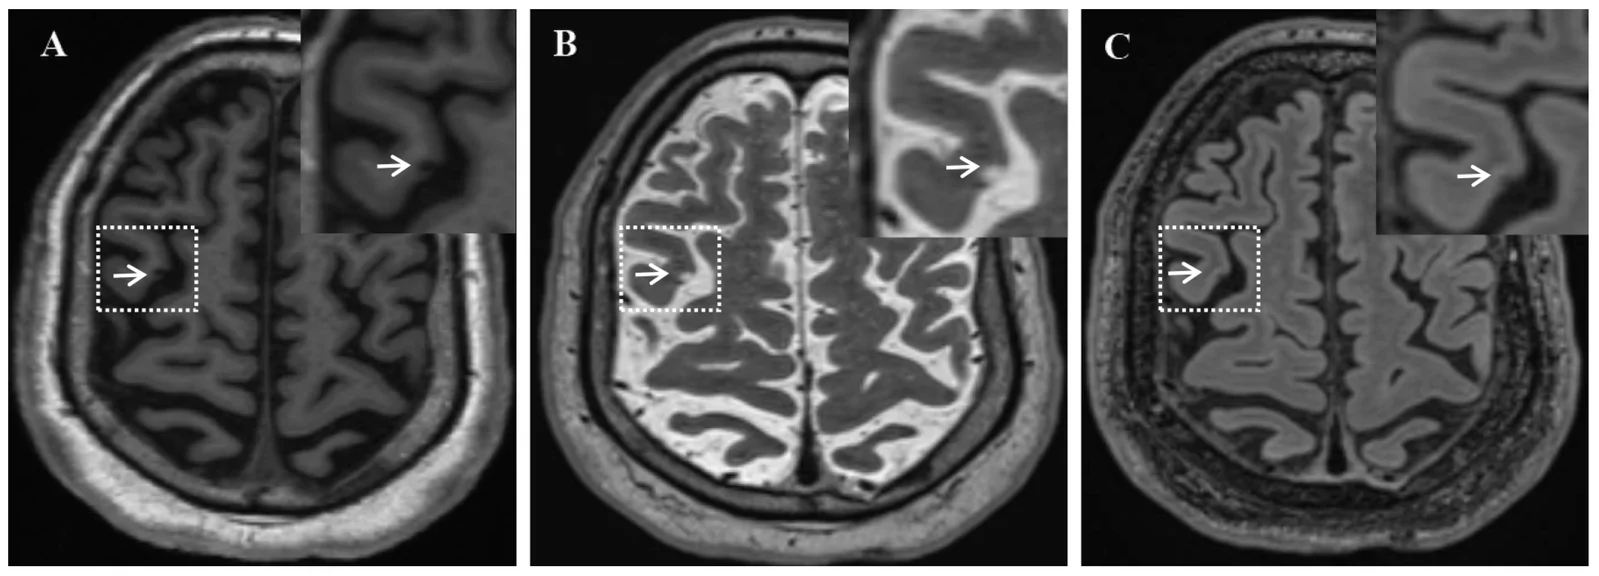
Example of a Cortical Cerebral Microinfarct on 3T MRI. White arrows indicate a cortical cerebral microinfarct appearing hypointense on T1-weighted imaging (A), hyperintense on T2-weighted imaging (B), and isointense on fluid-attenuated inversion recovery imaging relative to surrounding tissue (C). MRI: magnetic resonance imaging.
